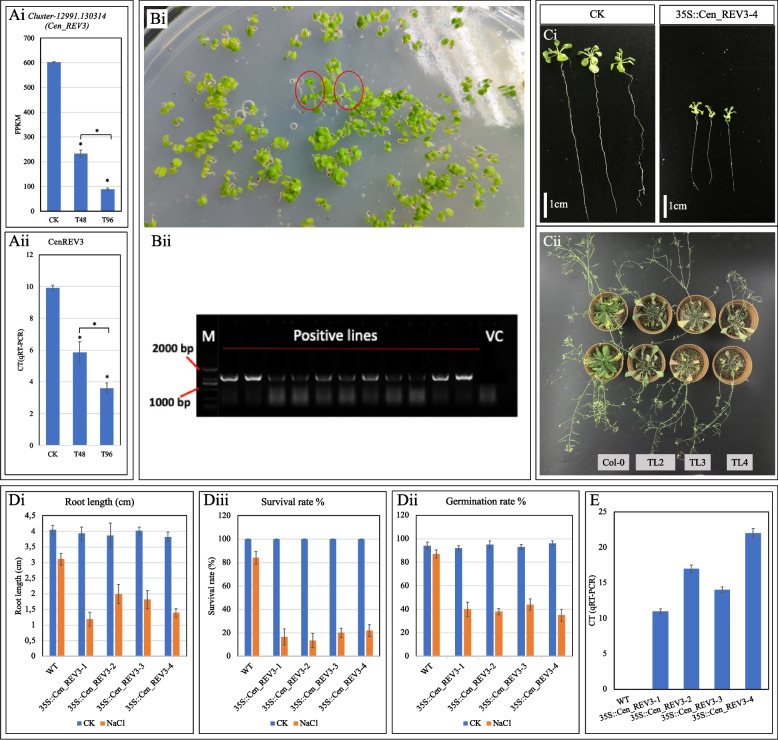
Salt stress tolerance analyses of the *CenREV3 Arabidopsis* overexpression lines. Ai) Expression (FPKM) and Aii) CT (qRT-PCR) of *CenREV3* in *C. ensifolium* leaves at CK, T48, and T96. The values are the mean ± SD of five replicates (*p* < 0.05). The asterisk sign (*) represents significant differences. B) Selection and confirmation of transgenic *Arabidopsis* lines. Bi) Transgenic lines selected on selection medium. Bii) Confirmation of positive transgenic *Arabidopsis* lines by PCR, where M = ladder, 2–11 wells are PCR confirmation results, and the last well represents vector control. Ci) Comparison of wild type and transgenic *Arabidopsis* seedlings under salt stress conditions (100 mM NaCl) for seven days. Cii) Morphological comparison of transgenic lines (TL) and wild-type after salt stress treatment (100 mM NaCl). Two rows present two different plants for the same line. C) Morphological analysis of the *CenREV3* overexpression lines; Di) root length, Dii) germination %, and Diii) Survival %. E) Expression (CT (qRT-PCR) of *CenREV3* in four transgenic lines. The qRT-PCR was repeated five times and the means were presented. Original pictures are presented in Supplementary data 1
